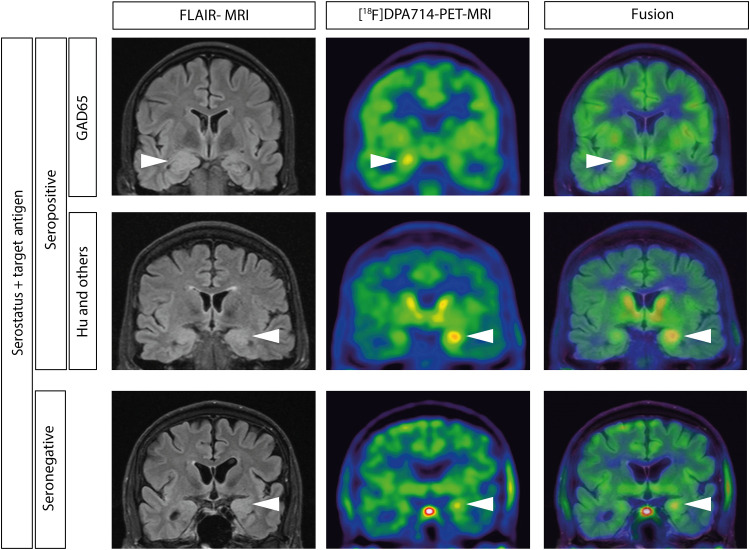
[^18^F]DPA-714 PET-MRI of patients with autoimmune limbic encephalitis. FLAIR-MRI (left), [^18^F]DPA-714 PET (middle), and fused (right) images of three representative patients with seropositive and seronegative ALE [one case of ALE with anti-GAD65 AABs (top), one case of ALE with anti-Hu AABs (and other AABs targeting intracellular neural antigens; center), and one case of ALE without detectable AABs (bottom)]. Focal uptake in amygdala/hippocampus of the affected hemisphere is marked with an arrow.
